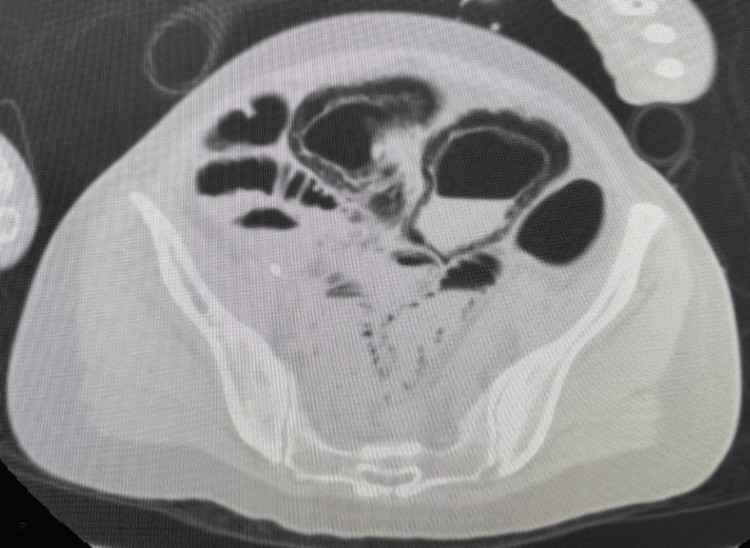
Abdominal computed tomography (CT) scan The CT scan showed no significant findings in the head; however, in the trunk, portal gas and intestinal dilatation from the jejunum to the sigmoid colon and intramural emphysema were observed.
